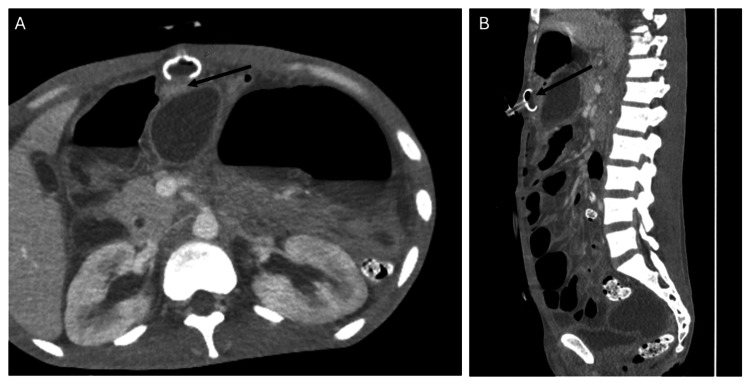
Malposition of a Percutaneous Gastrostomy Tube With Intraperitoneal Placement Non-contrast CT of the Abdomen Demonstrating Malposition of a PEG Tube (A) Axial view shows the tube situated anteriorly, outside the gastric lumen (arrow highlights the gap). (B) Sagittal reconstruction confirms extragastric location of the tube (arrow highlights the gap), with peritoneal enhancement and free fluid suggesting peritonitis from continued tube use. Early recognition of extragastric placement is critical to prevent ongoing leakage, sepsis, and other complications.
